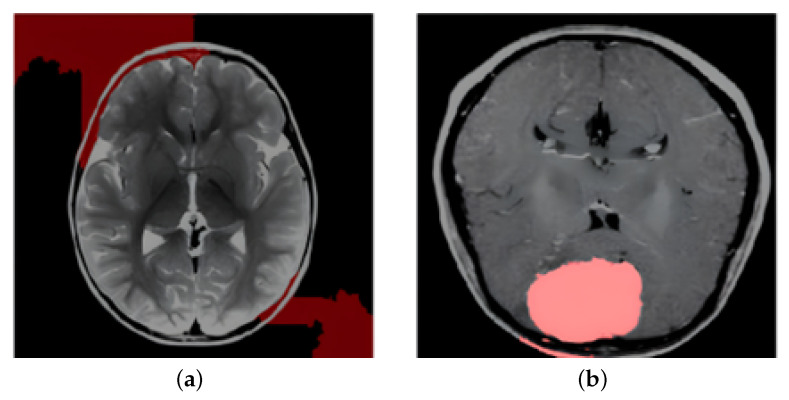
LIME-based local interpretability of model predictions on brain MRI scans: (a) LIME explanation for a non-tumor case (sample 0) showing sparse and low-importance regions, indicating that the model’s prediction is not influenced by irrelevant features and (b) LIME output for a tumor case (pred 4) where the tumor region is prominently highlighted as the most influential factor in the model’s classification decision, confirming alignment with human clinical reasoning.
